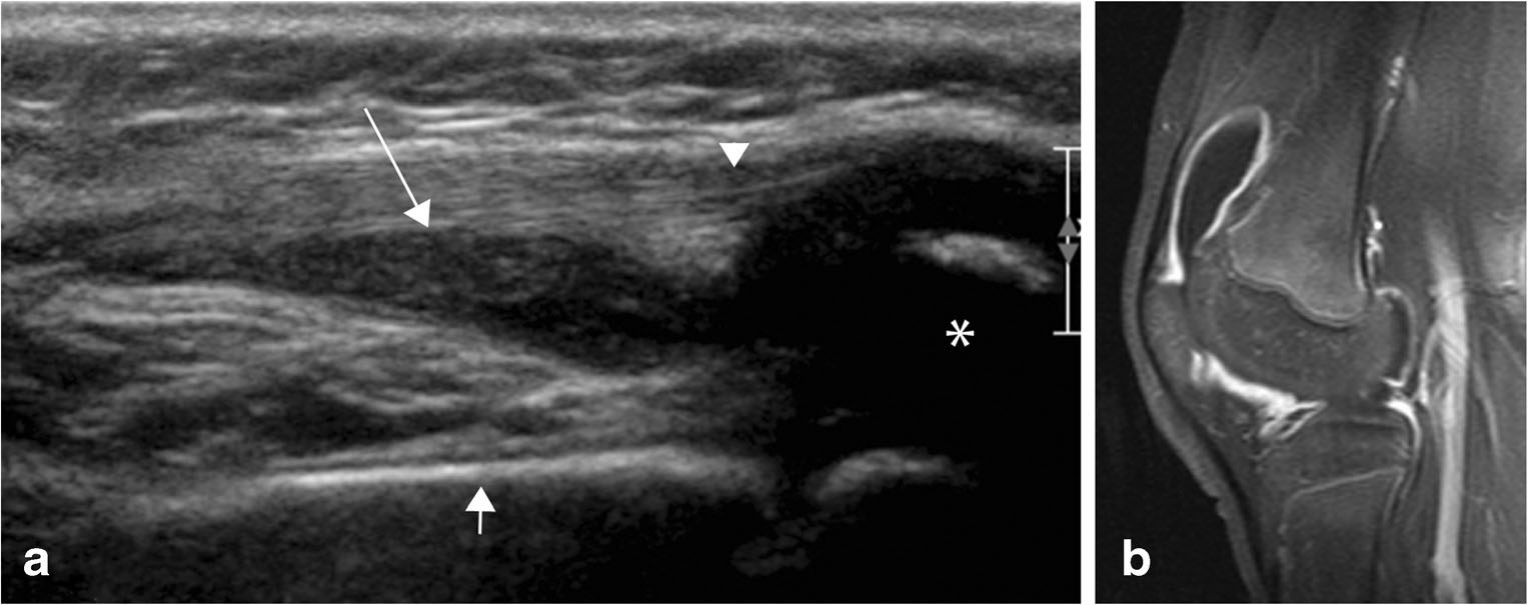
a Ultrasound (panoramic view) of the ventral aspect of the knee shows fluid in the supra-patellar recess (arrow), cranial to the patella (asterisk), under the patellar tendon (arrowhead) and overlying the femur (small arrow) suggestive of synovitis. b The findings were confirmed by MRI, which shows slightly hypertrophic, enhancing synovium in keeping with synovitis
